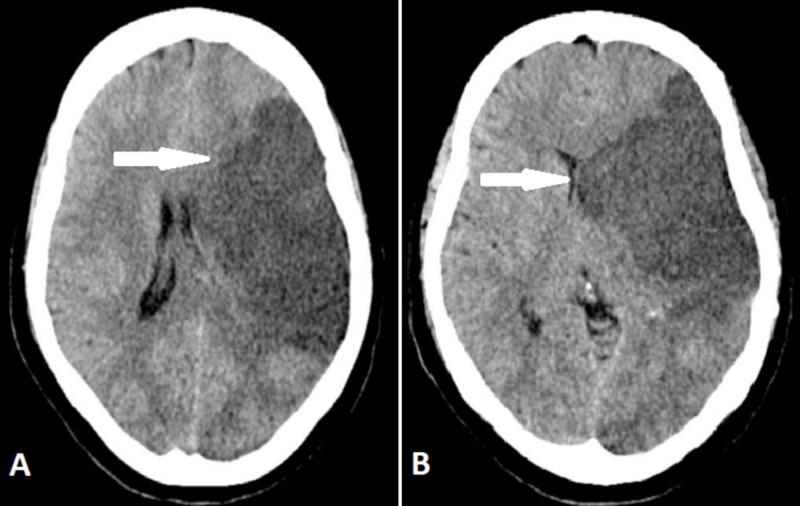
CT scan of head These are CT scans of the head without contrast in axial view showing hypodense area in the left middle cerebral artery territory
